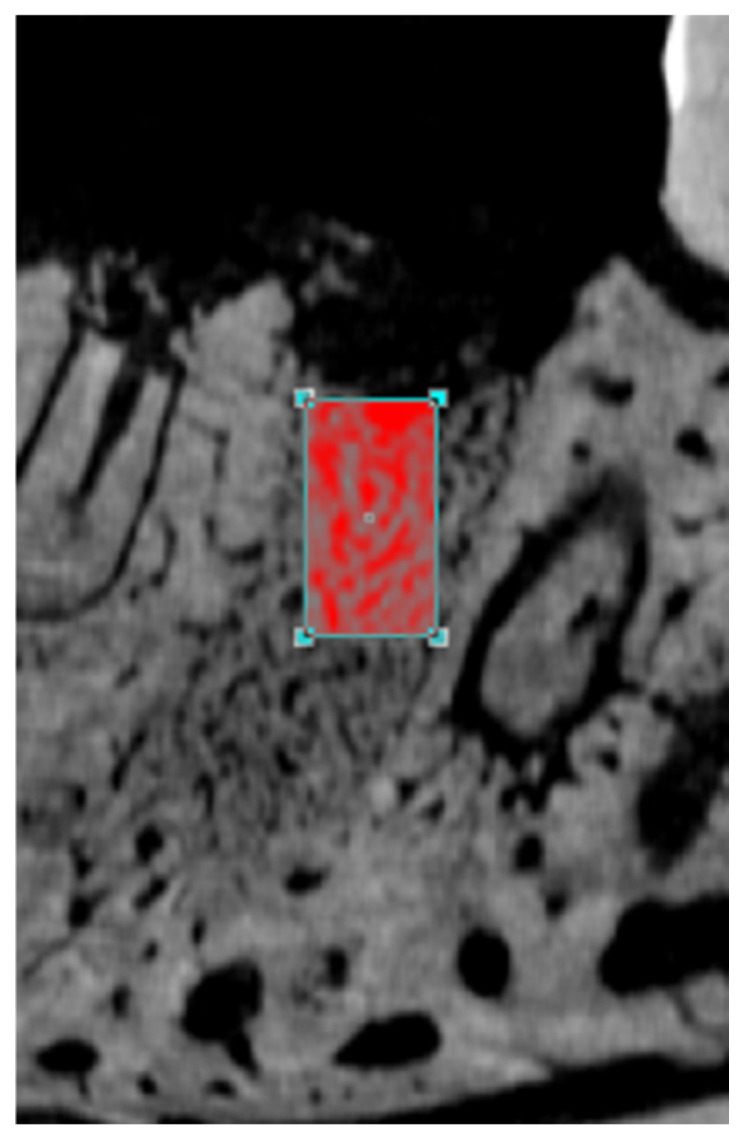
The radiographic area of interest (ARI) was defined as a 4 mm^3^ region at the site of the lower left first molar extraction.
